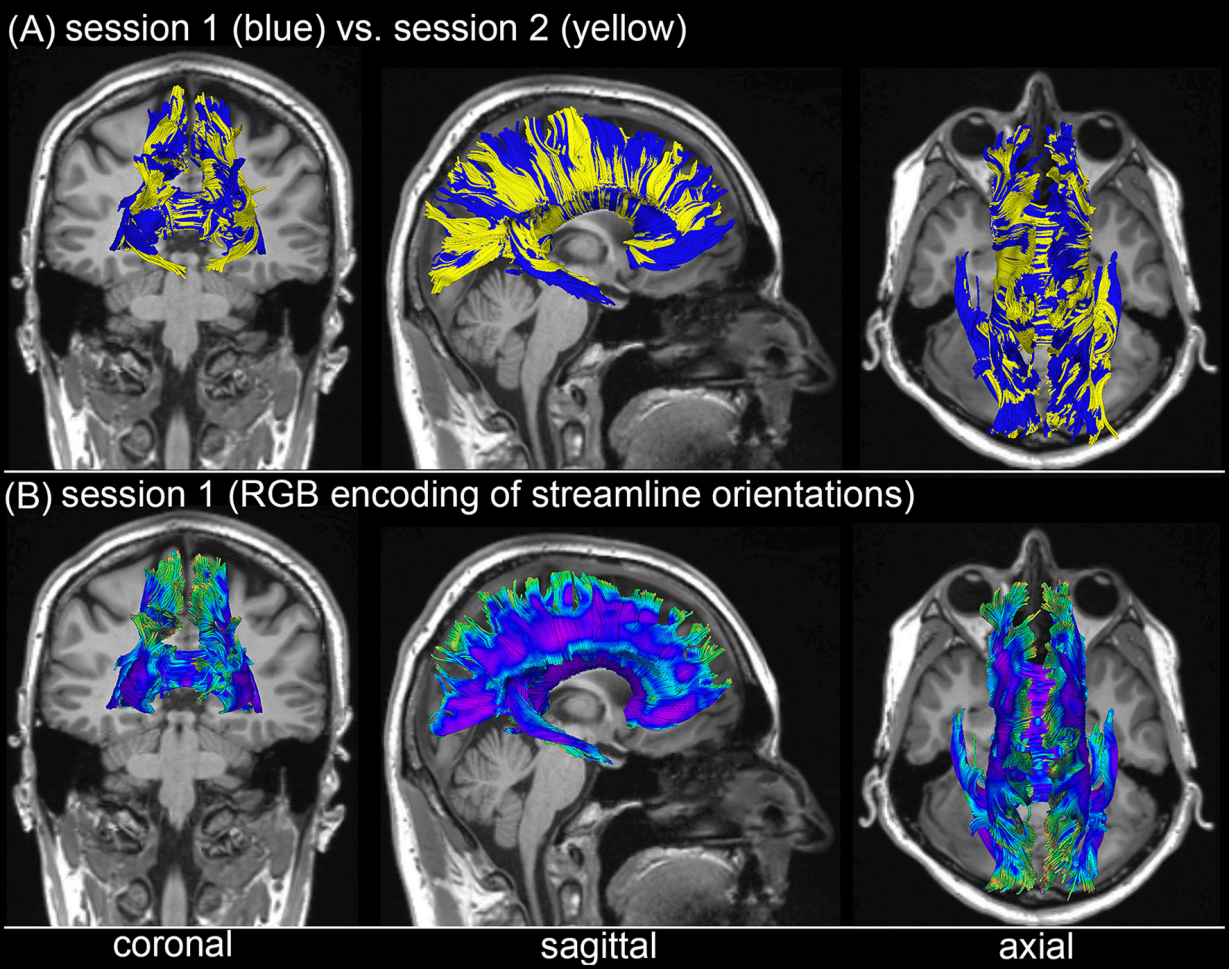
Depiction of DTI-derived streamlines in the corpus callosum superimposed on the *T*_1_-weighted MRI scans of a sample HC volunteer. (A) Two sets of streamlines (blue, yellow) were reconstructed based on scans acquired during distinct sessions. Despite similarities between the two sets of streamline bundles, noticeable differences abound. (B) When displayed using red-green-blue (RGB) encoding of streamline orientations, the DTI streamlines derived from scans acquired during session 1 are consistent with the expected neuroanatomy of the healthy corpus callosum.
